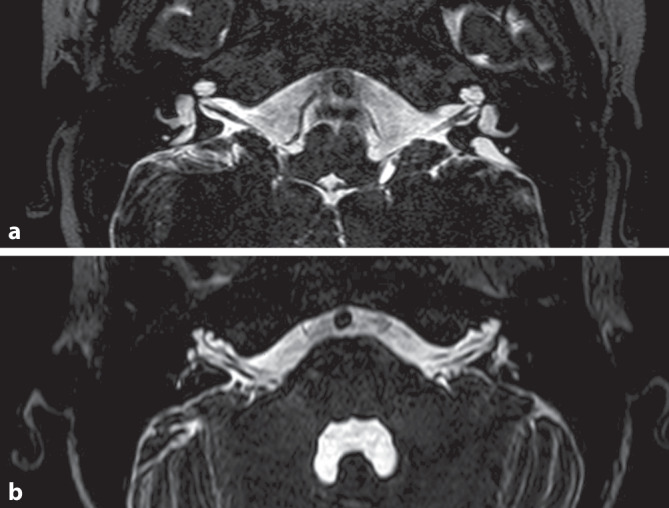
T2-weighted magnetic resonance imaging of the cerebellopontine angle in two patients (a, b). Axial plane. (From [27] © S. Rösch et al., CC BY 4.0, https://creativecommons.org/licenses/by/4.0/). a Patient with a clearly enlarged vestibular aqueduct on both sides, dorsal to the horizontal semicircular canal. b Patient with bilateral X‑linked malformation of the cochlea with a bilateral missing modiolus (“incomplete partition type III”)
